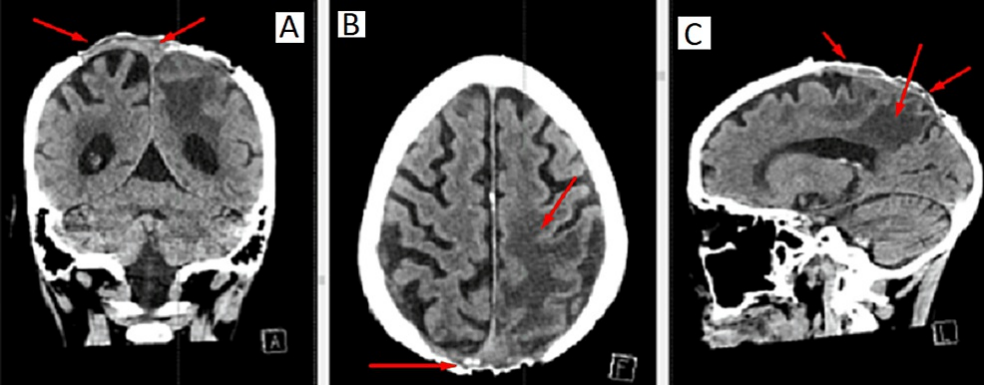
Preoperative CT scan showing evidence of invasion of an extra-axial neoplastic process in both parietal bones and subdural space. Vasogenic edema in the left and right parietal lobes. A: Coronal plane. B: Axial plane. C: Sagittal plane.
